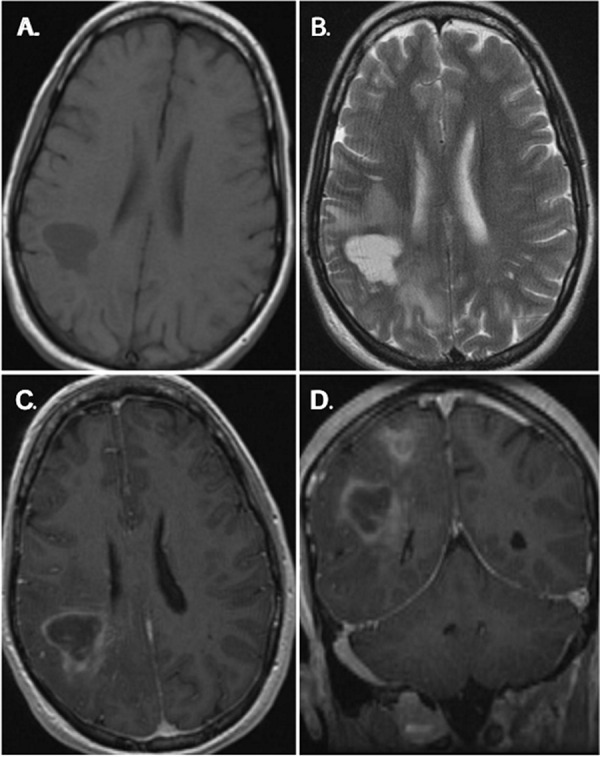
Magnetic resonance imaging (MRI) findings. Brain MRI demonstrates two irregularities. The primary lesion was centered in the posterior-inferior parietal lobe (A, B, C) and a second contiguous lesion was identified in the posterior-central parietal lobe (D). The lesions were T1-hypointense (A) and T2-hyperintense (B) with peripheral irregular enhancement on T1 imaging following administration of gadolinium (C, D). Abnormal T2/FLAIR hyperintense signal surrounded both lesions.
